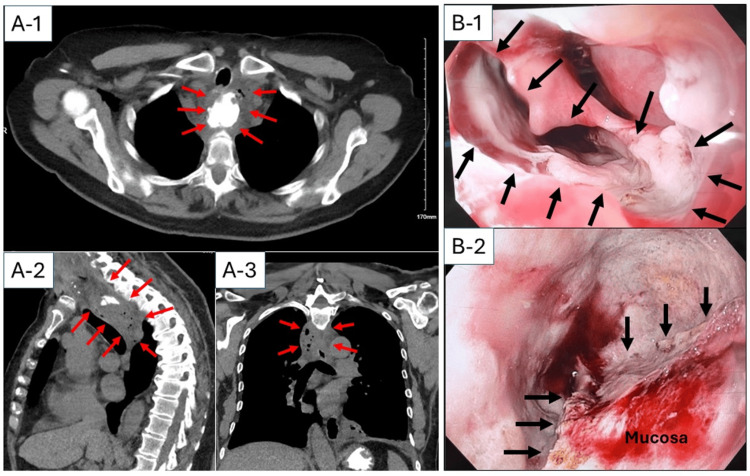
Computed tomography (CT) and endoscopic findings on postoperative day 5 (A-1) axial, (A-2) sagittal, and (A-3) frontal views of CT scan  demonstrated a 4.3 x 3.3 x 7.8 cm contained fluid collection with gas formation posterior to the proximal esophagus (red arrows). (B) EGD revealed a 15 x 10 mm complete mucosal dehiscence (B-1, arrows) with 4 cm deep contained cavity posterior to the esophagus (B-2, arrows indicate the edge of myotomy).
